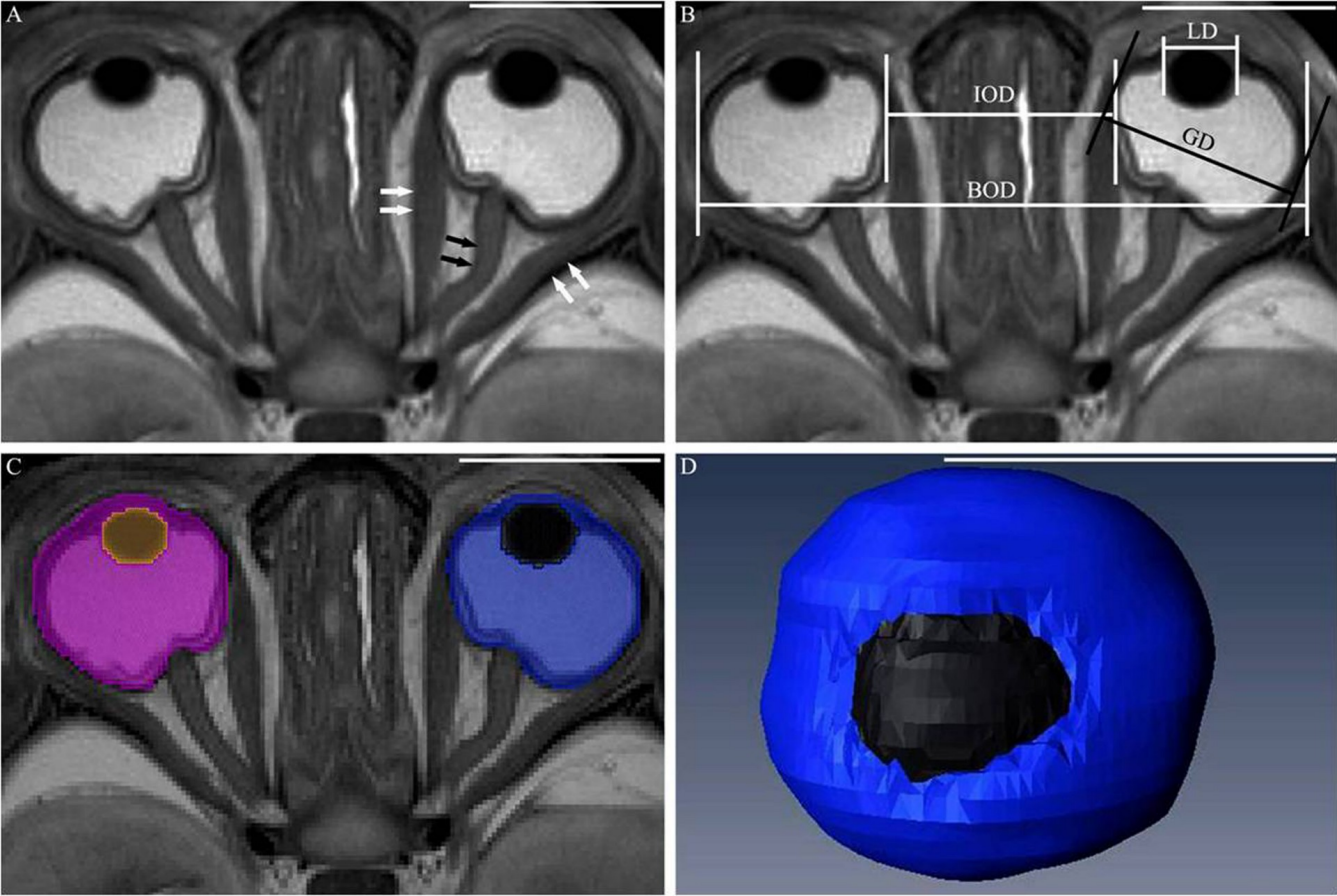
Transverse T2-weighted 7.0 T MRI scans of the fetal ocular structures of 20 weeks GA (A) and their linear measurements (B), manual segmentation (C) and a 3D visualization model of the eyeball (D). The lens, vitreum, three layers of the eyeball wall, optic nerve (black arrows) and ocular muscles (white arrows) can be clearly delineated (A). B demonstrates measurements of the IOD, BOD, GD and LD. The eyeball is divided into lens and other structures, and was filled with different colors (C). D is the 3D visualization model of the eyeball and can dynamically demonstrate the eyeball. The bar in each figure represents 1 cm.
